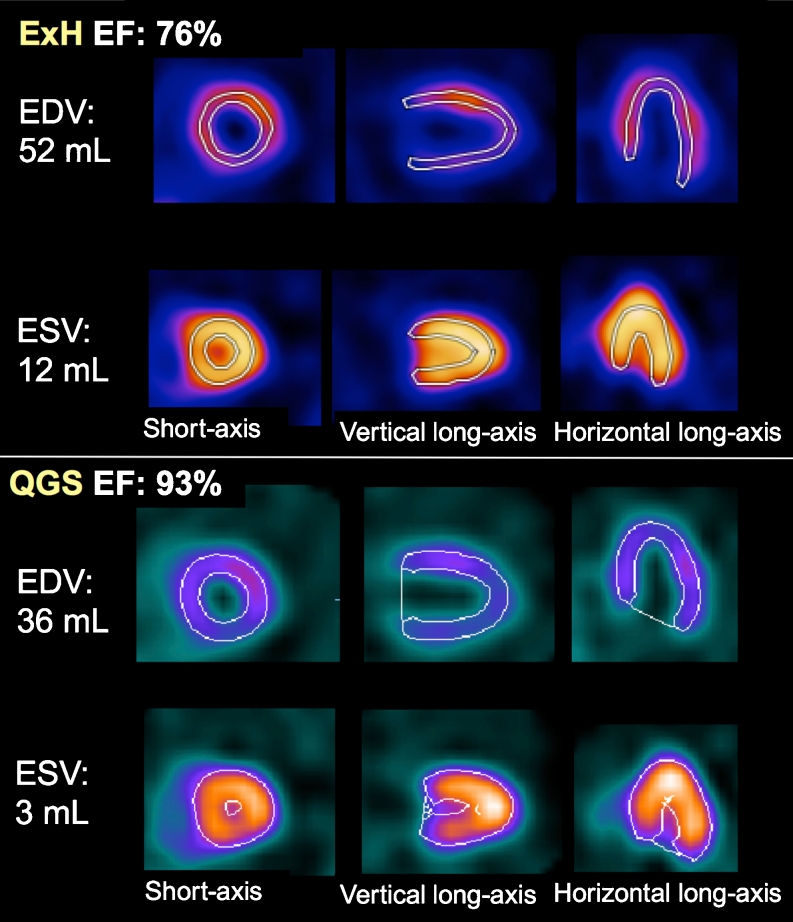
Preoperative gated SPECT imaging in a A 78-year-old woman with a small heart (height 142 cm, weight 43 kg, BSA 1.17 m^2^). The EFs calculated using ExH and QGS were 76 % and 93 %, respectively. Echocardiography showed EDV 64 mL, ESV 15 mL and EF 77 % by Teichholz’s formula
